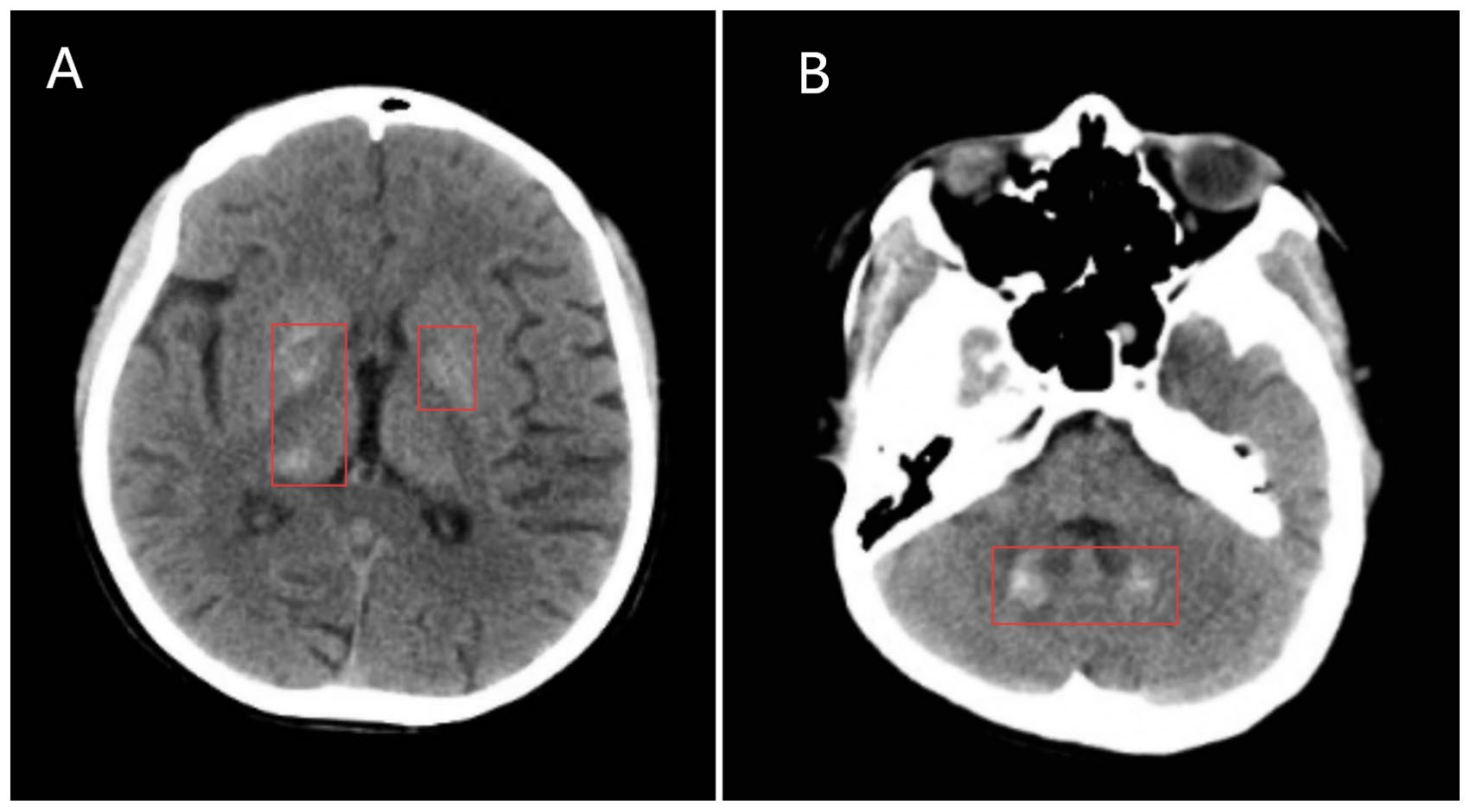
Head CT. (A) High-density lesions in the bilateral basal ganglia (red mark). (B) High-density lesions in the cerebellar dentate nuclei (red mark).
